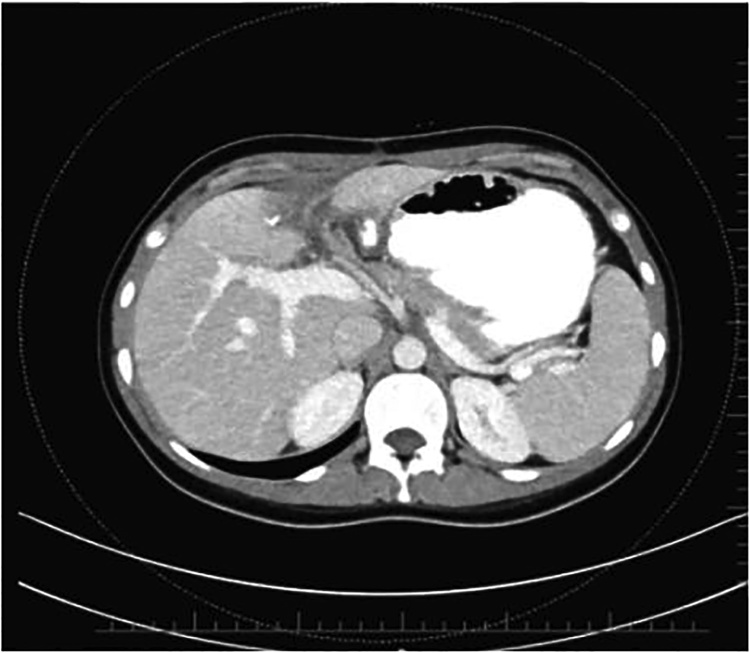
Computed tomographic follow-up image 10 months after surgery.
